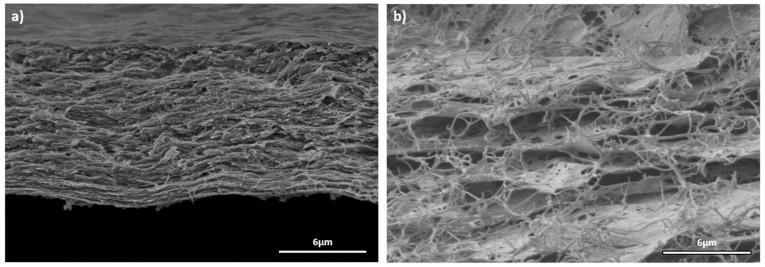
SEM images of (a) KBC-CT and (b) KBC-L.
